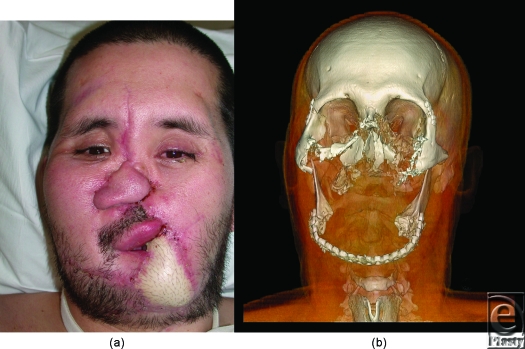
(*a*) Candidate 2 during initial evaluation for face transplantation. (*b*) 3-dimensional volume rendering from the computed tomographic acquisition with windowing to show the bony defects that must be considered in surgical planning.
